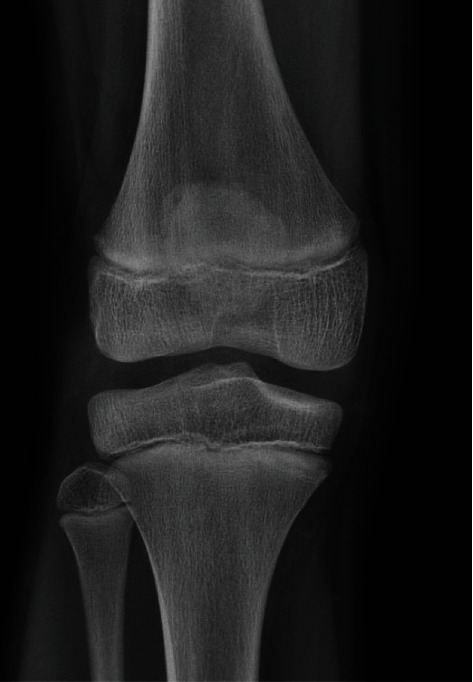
X-ray 5. AP view of the right knee, 6 weeks after diagnosis.
